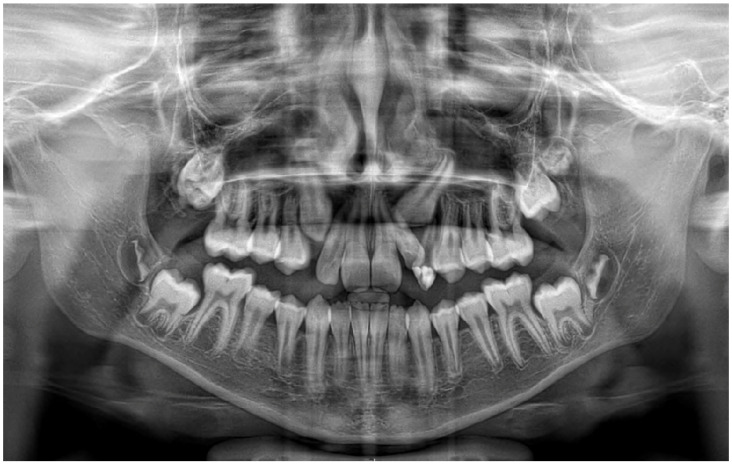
A three-year follow-up panoramic radiograph showing no recurrence of the lesions.
